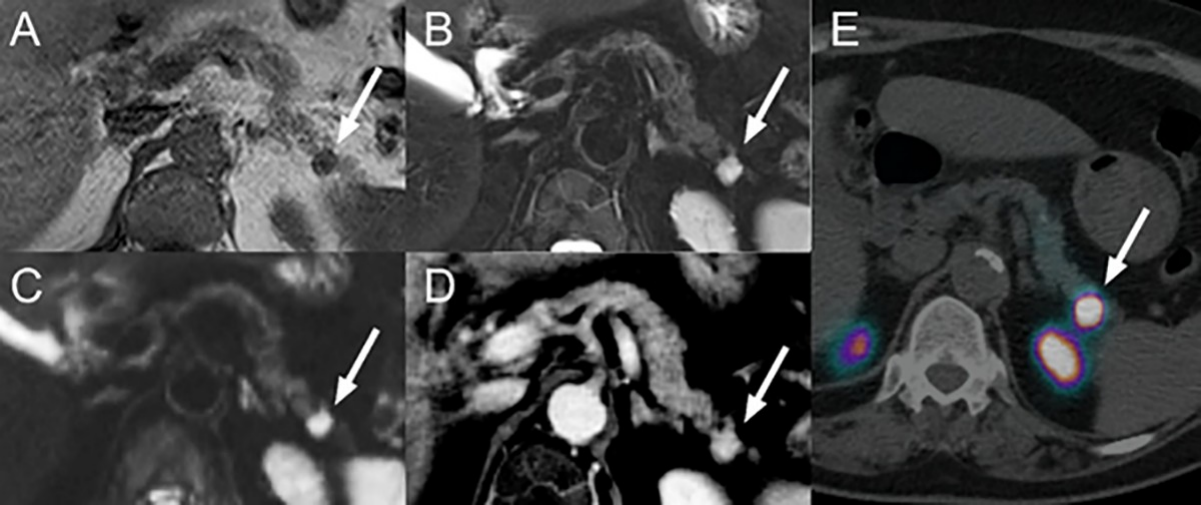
Imaging example of a small PICT with typical signal characteristics. PICT localized in the pancreatic tail (white arrow): hypointense on in-phase T1w (A), hyperintense on T2w fs T2w BLADE (B), hyperintense on DWI b-value 800 s/mm^2^ (C) and hyperenhancing on ce T1w Dixon VIBE (D). ^68^Ga-DOTA-exendin-4 PET/CT (E) confirming the lesion.
